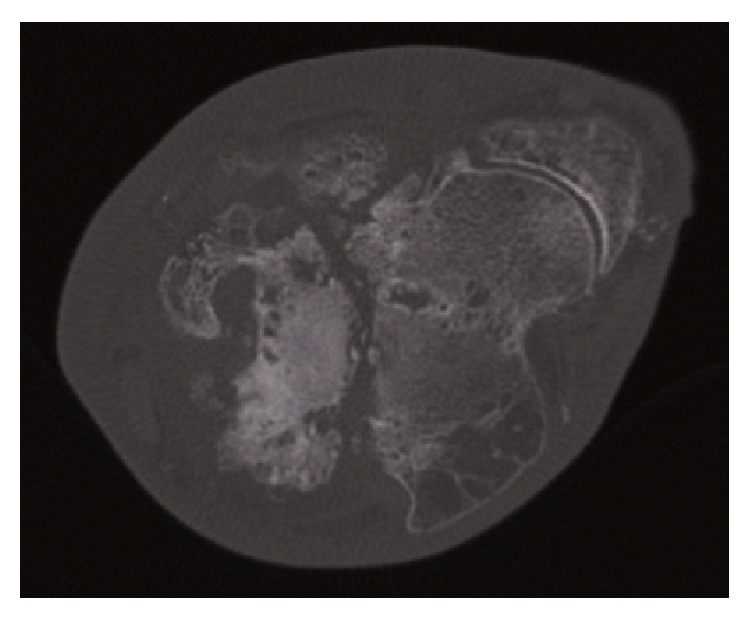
CT scan at presentation: axial slices demonstrate size of distal fragment.
